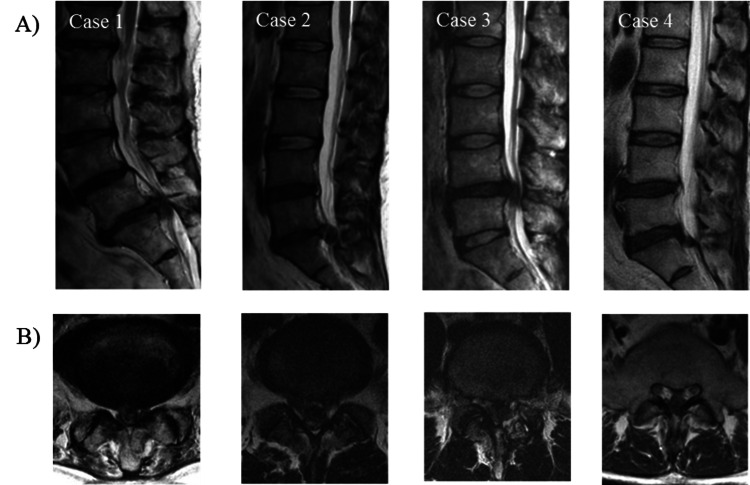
Sagittal and axial magnetic resonance imaging showing lumbar disc herniation of four cases. (A) Sagittal view. (B) Axial view.
